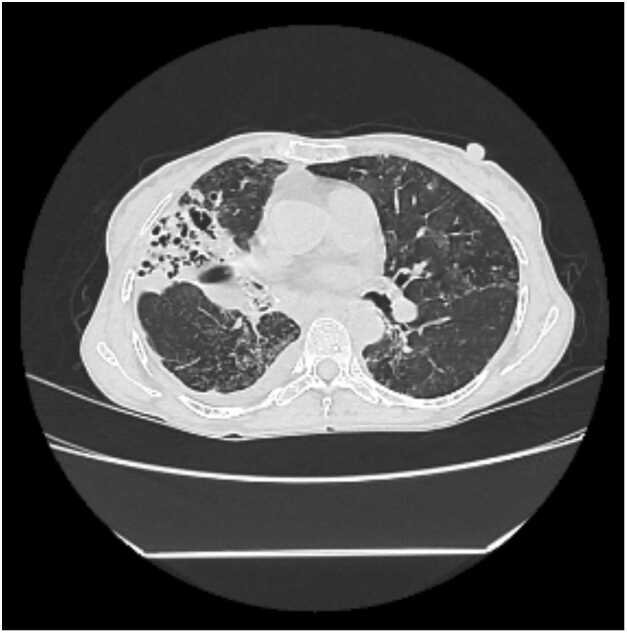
High-resolution contrast-enhanced computed tomography (CECT) of the thorax.
